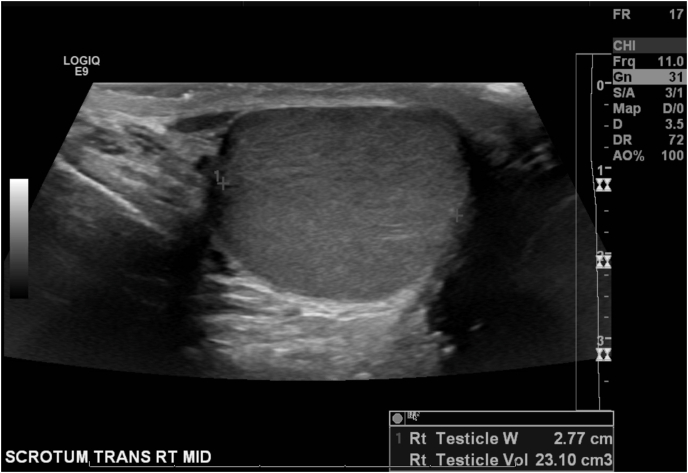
Ultrasound of right testicle
